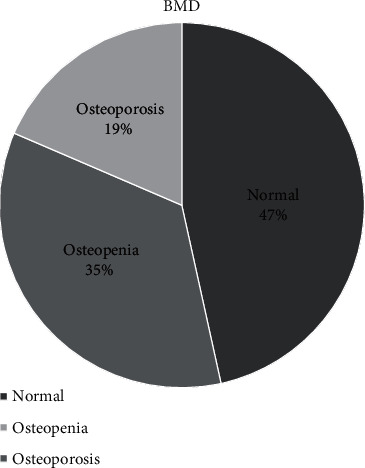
Prevalence of osteoporosis and osteopenia amongst male patients (*n* = 507) who underwent CT scans at Kalafong Hospital, South Africa.
